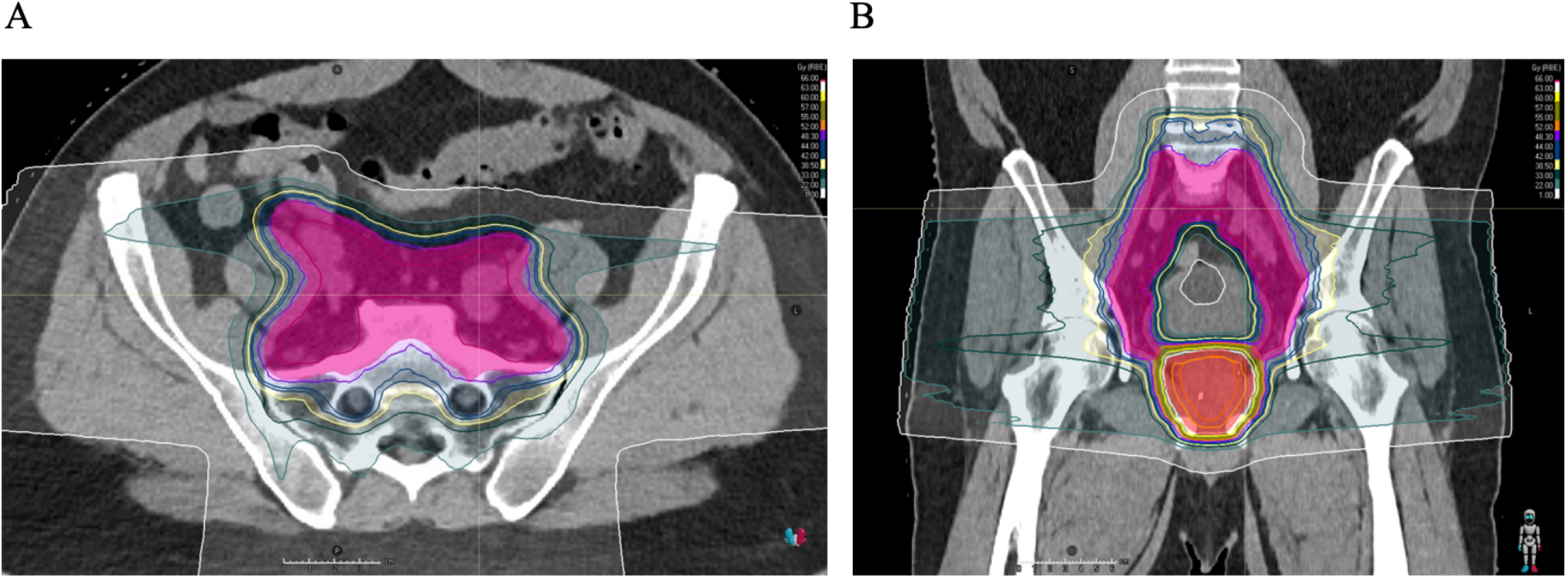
Transversal (A) and coronal (B) CT slices overlaid with a colourwash representation of the dose distribution for the treatment of prostate cancer with pelvic lymph node irradiation. High dose of 63.0 Gy to PTV of the prostate (shaded red), lower dose of 48.3 Gy to PTV of the lymph nodes and seminal vesicles (shaded pink, seminal vesicles not seen in these sections). Treatment planning done using the Raystation^®^ treatment planning system (RaySearch Laboratories AB).
